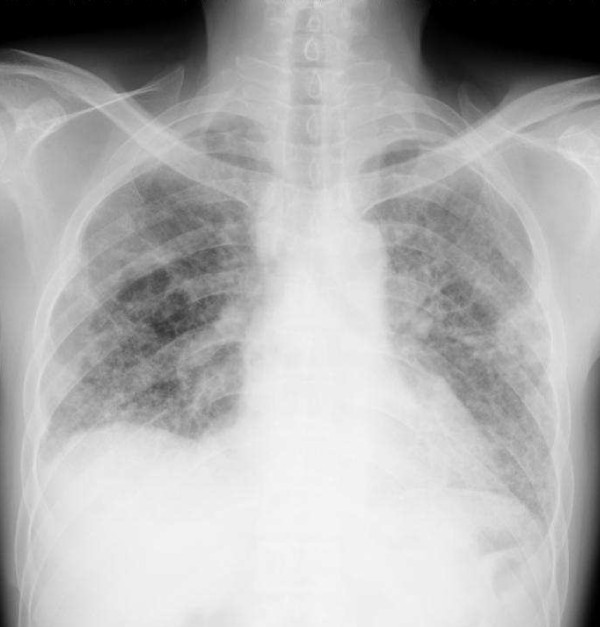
Chest radiography four weeks after treatment reveals improvement of pneumothorax and pulmonary infiltration.
